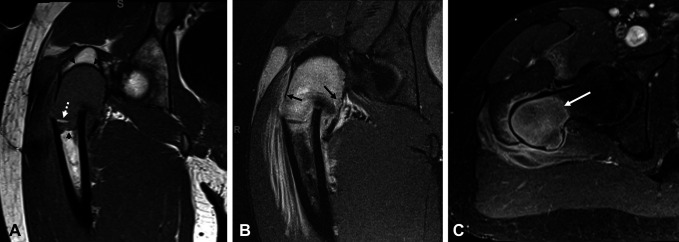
MRI of the right hip with and without contrast: A, Coronal
